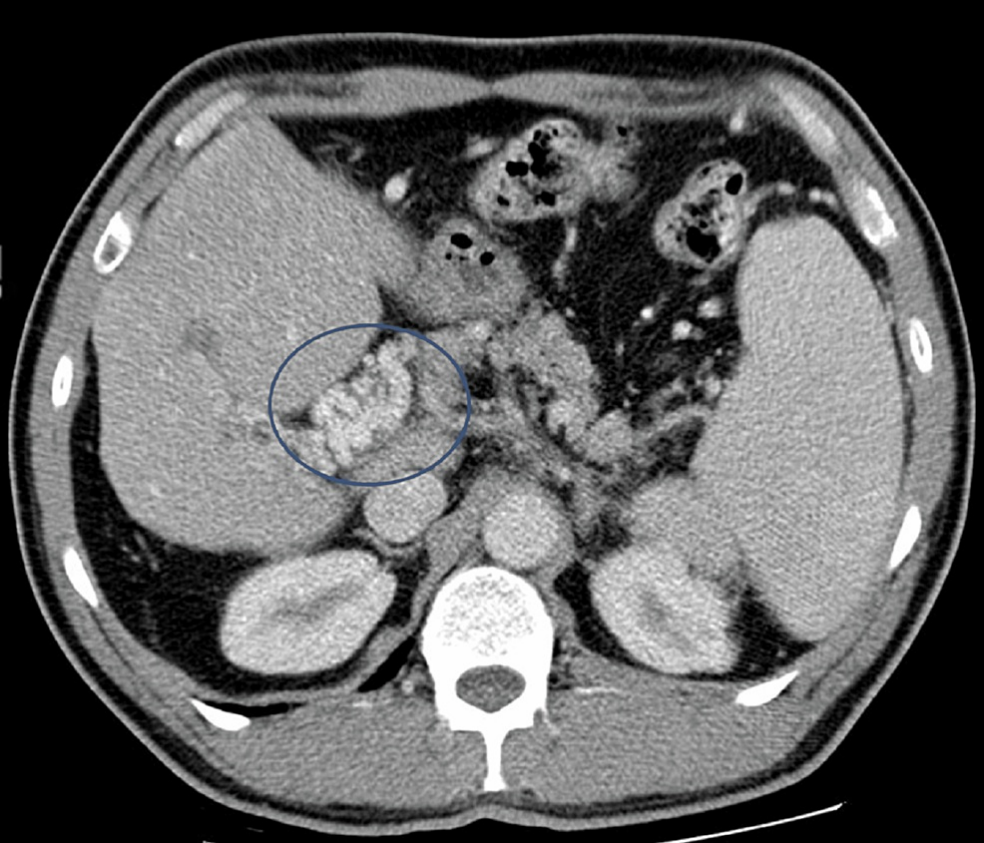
Abdominopelvic CT with intravenous contrast The total transformation into portal vein cavernoma (circle).
